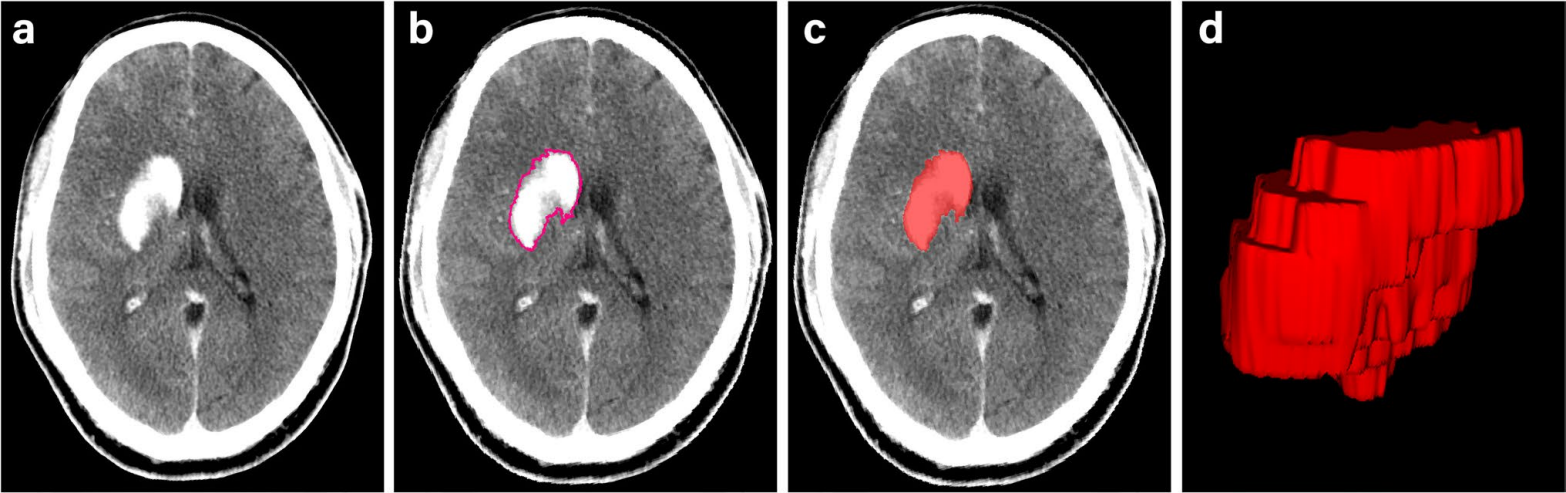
Delineation of the hyperattenuating areas using ITK-SNAP software. a A hyperattenuating area in the left lentiform nucleus on nonenhanced CT. b Manual segmentation along the hyperattenuating area contour on the transverse section. c The ROI of the hyperattenuating area on a transverse section is displayed as the red area. d 3D ROI for the whole hyperattenuating area. 3D three-dimensional, CT computed tomography, ROI region of interest
